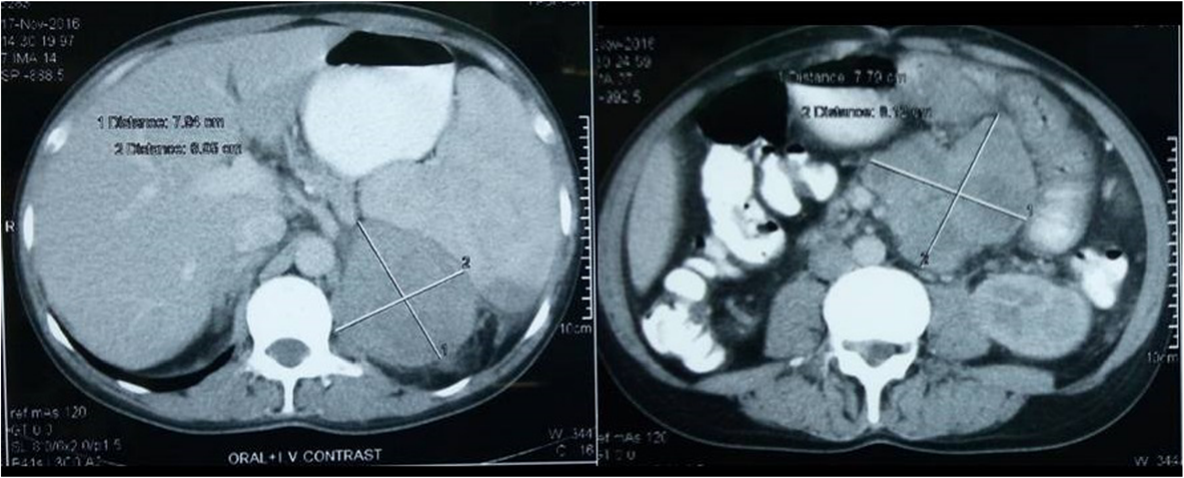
Contrast-enhanced CT scan of the abdomen (Left) showing lesion in the left upper abdomen posterior to the spleen and superior to the left kidney (right) showing matted mass in small bowel mesentery
